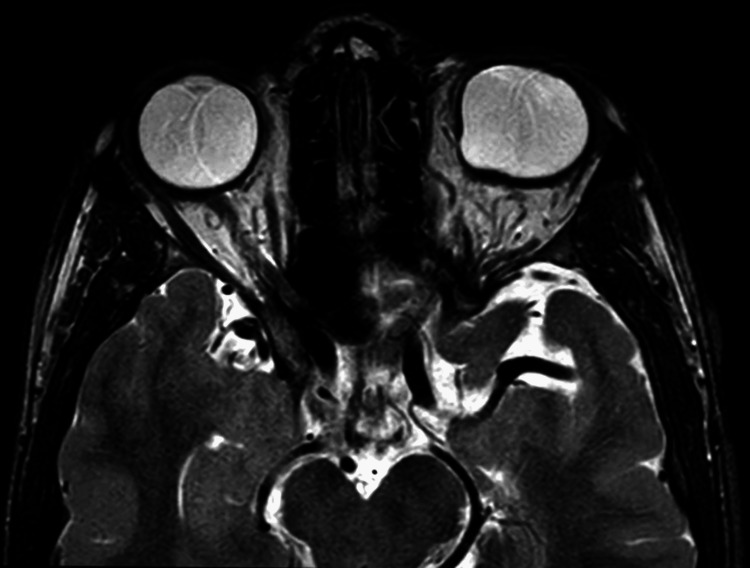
MRI-axial section T2WI of bilateral orbit suggestive of posterior staphyloma in the left globe
